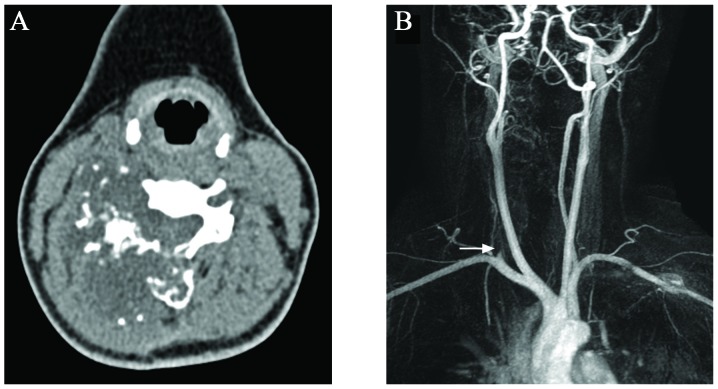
(A) Axial CT scan of the C5 vertebra. There is a defined lytic lesion and a large soft-tissue mass within the mottled calcification, and the tumors that were involved in the processus transversus, lamina and spinous process are on the right. (B) Angiography showing a lack of blood flow in the right vertebral artery (white arrow) and compression of the common carotid artery. CT, computed tomography.
